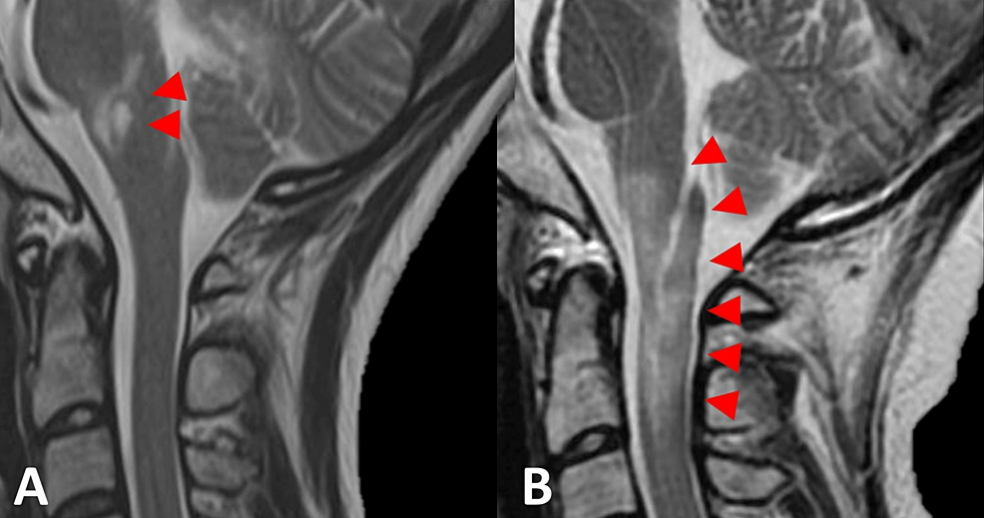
Cervical MRI T2WI at first and second attack Cervical MRI showed T2WI high signal at the level of medulla level (A) at the first attack, and cervical MRI revealed T2WI hyperintense lesions from the medulla to the cervical vertebral bodies 2 level in the spinal cord at the second attack. T2WI: T2-weighted imaging
